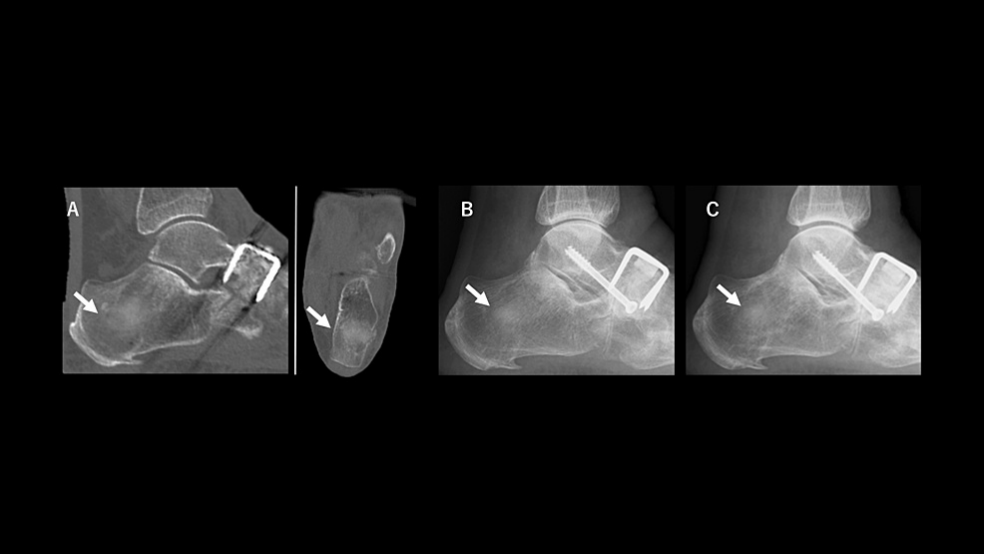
Postoperative radiograph and computed tomography (CT) of the foot (26 days~) (A): Sagittal and transverse views of CT analysis at 26 days after surgery. Transposition to host bone trabeculae was almost complete. Talonavicular joint fusion also seems to be complete. The white arrow indicates the site of calcaneal bone harvesting, filled with newly formed bone. (B), (C): There is no problem at the harvesting site one month (B) and three months (C) after surgery. The talus dorsiflexion correction was completed. The white arrow indicates the site of calcaneal bone harvesting, filled with newly formed bone, and continuation with the host bone trabeculae was also observed.
